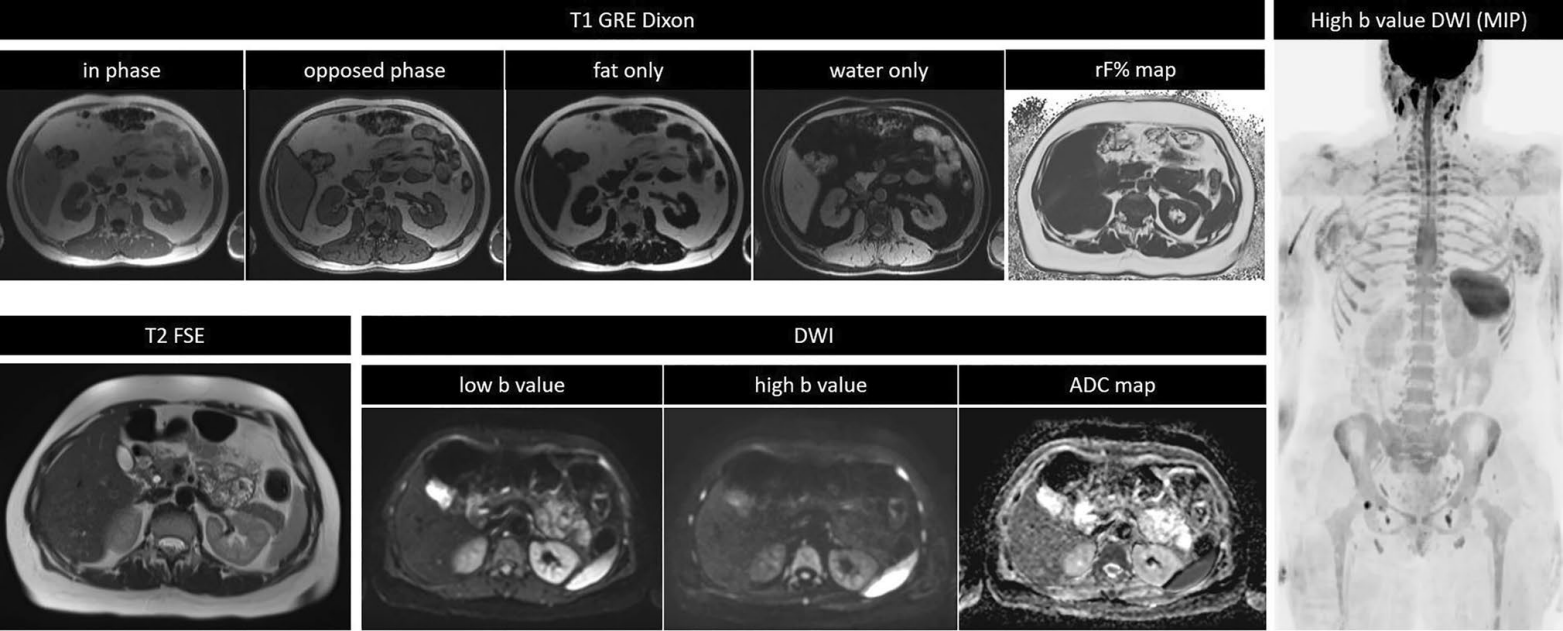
Core protocol. This image summarizes the main pulse sequences included in the WB-MRI protocol for cancer screening. The study should cover from head to pelvis. T1 weighted images are acquired with a gradient recalled echo (GRE) sequence, preferably with Dixon technique, to obtain four different sets of images (in-phase, opposed-phase, water-only, fat-only) and to allow the calculation of the relative fat-fraction (rF%) map. T2 images are acquired with a single-shot fast spin echo (FSE) sequence. Diffusion-weighted images (DWI) should be acquired with at least two b values in order to obtain the corresponding apparent diffusion coefficient (ADC) map. Maximum intensity projections (MIP) should be reconstructed from the highest b value DWI images
